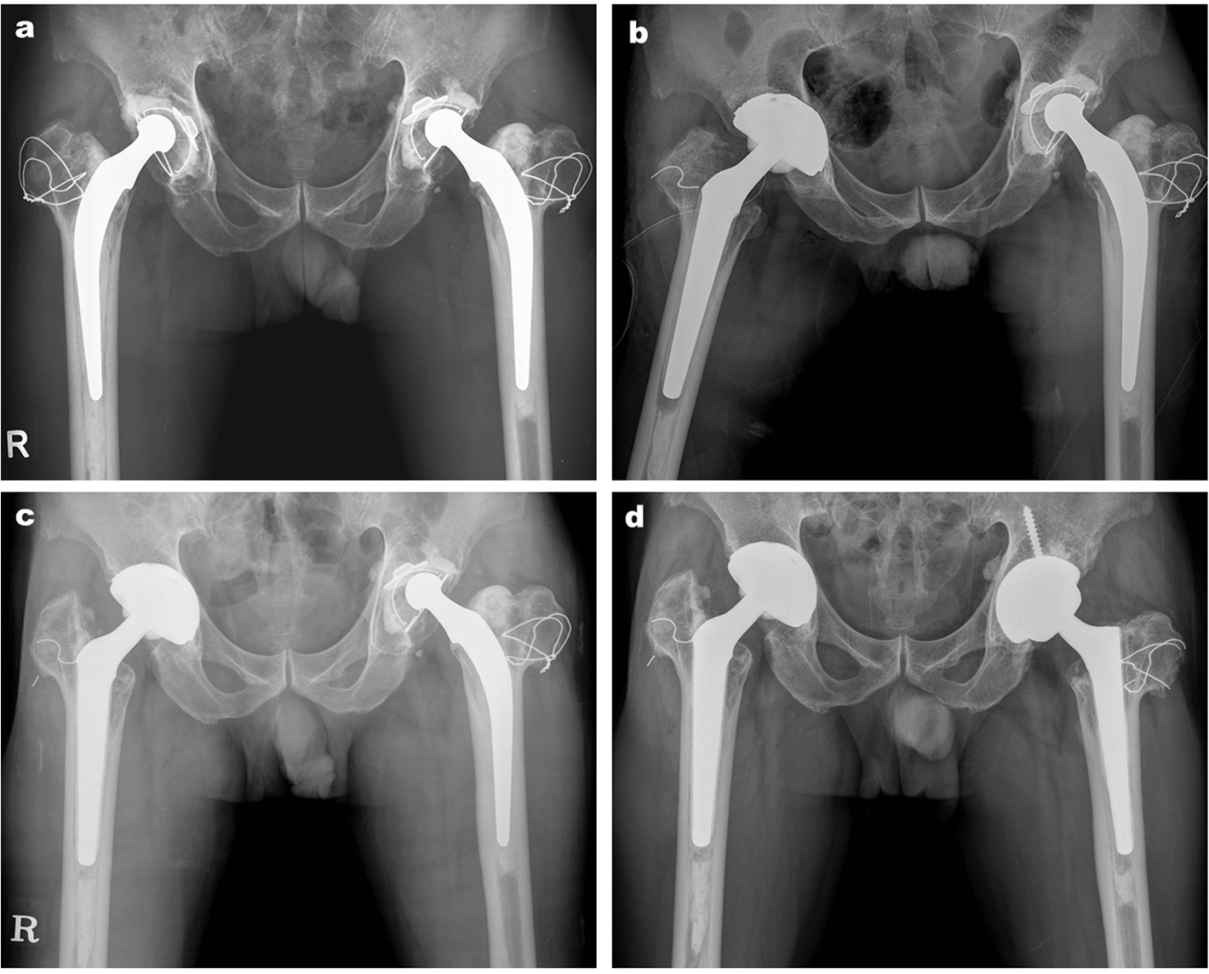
a Preoperative radiograph of a 66-year-old male with aseptic loosening of the cemented cup and well-fixed Charnley femoral stem. b Immediate postoperative radiograph: revision was performed using the Wagner cone prosthesis and cementless acetabular cup without removing the distal part of the cement. c Anteroposterior radiograph of the hip 9 years postoperatively. d Anteroposterior radiograph of the hip 15 years postoperatively; the left hip was also revised using the Wagner cone prosthesis 6 years ago
